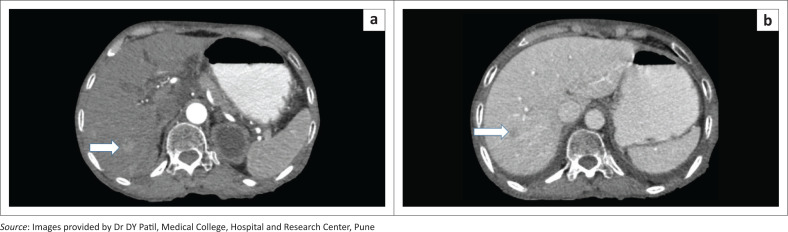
(a–b) Axial CT abdomen- arterial (a) and portal phase (b) revealing a hepatic metastasis (white arrow) in segment VI appearing hyperdense in the arterial and hypodense in the portal phase.
